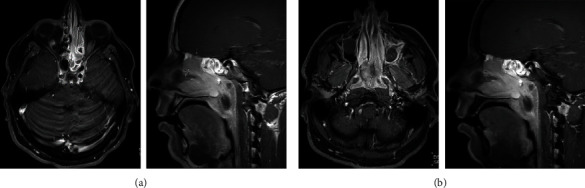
Contrast-enhanced T1 fat sat axial and sagittal MRI images showed two contrast-enhancing masses: one is nasoethmoidal (a), and the second one is in the nasal choana and postnasal space (b).
